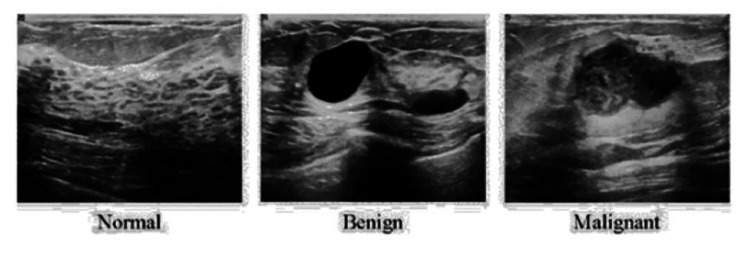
X-ray image of the breast and its cancer type Source: Reference [32]
